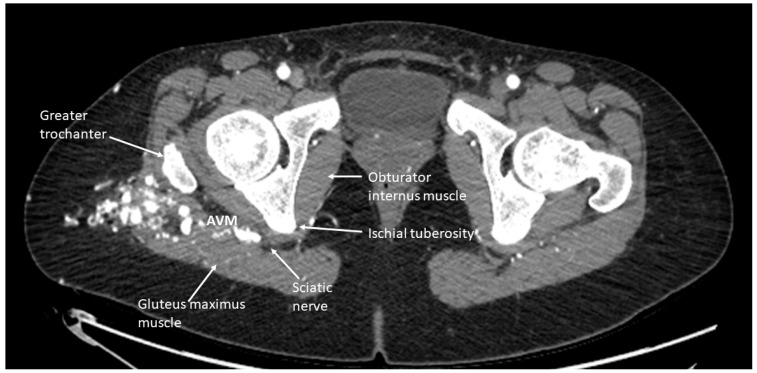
CT scan imaging of a right hip arteriovenous malformation with involvement close to the sciatic nerve. This patient had undergone several sclerotherapy procedures and received a quadratus lumborum nerve block for postoperative pain management. Following one therapy, the patient was noted to have a sciatic nerve injury.
